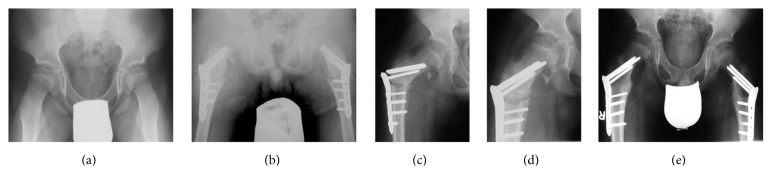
((a) and (b)) Anteroposterior preoperative (a) and postoperative (b) radiographs of a 10-year-old boy with infantile cerebral palsy with coxa antetorta who underwent femoral derotation osteotomy with use of a locking plate on both sides. (c) Anteroposterior radiograph of the same patient demonstrates persistent femoral nonunion with screw breakage of two femoral neck screws on the right side. (d) Anteroposterior radiograph two days after surgical revision with removal of the locking plate, resection of the femoral nonunion, application of rhBMP-2, and insertion of a new locking plate. (e) Anteroposterior radiograph 7.9 months after surgical intervention with use of rhBMP-2 demonstrating complete radiological healing which was still evident at the most recent follow-up, 60 months after rhBMP-2, was applied.
